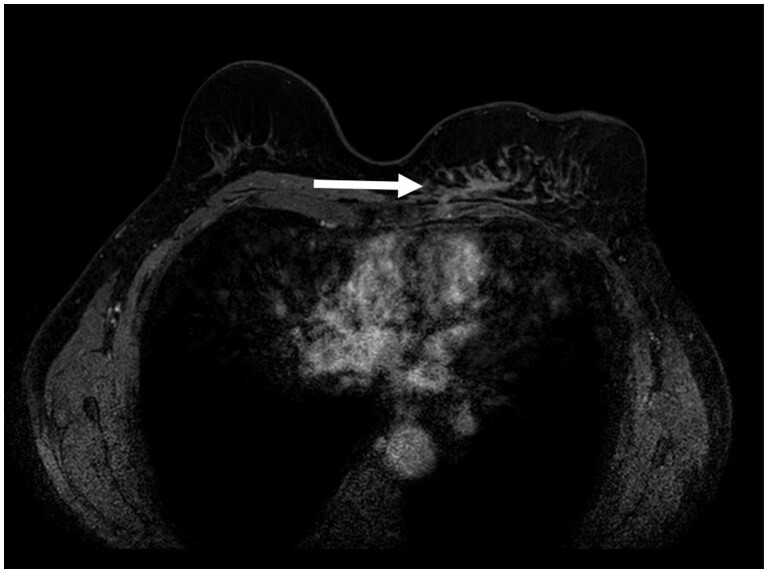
Breast MRI of a 64-year-old woman with a unilateral left-sided total breast reconstruction with AFT, because of a multifocal left-sided invasive carcinoma NST (ER+PR+Her2−) treated with mastectomy. Breast MRI demonstrates an enhancing mass prepectoral on the inner side of the reconstructed breast extending through the pectoral muscle (white arrow). After core-needle biopsy, recurrent breast cancer was confirmed (invasive carcinoma NST, ER+PR+Her2−). Abbreviation: AFT = autologous fat transfer.
